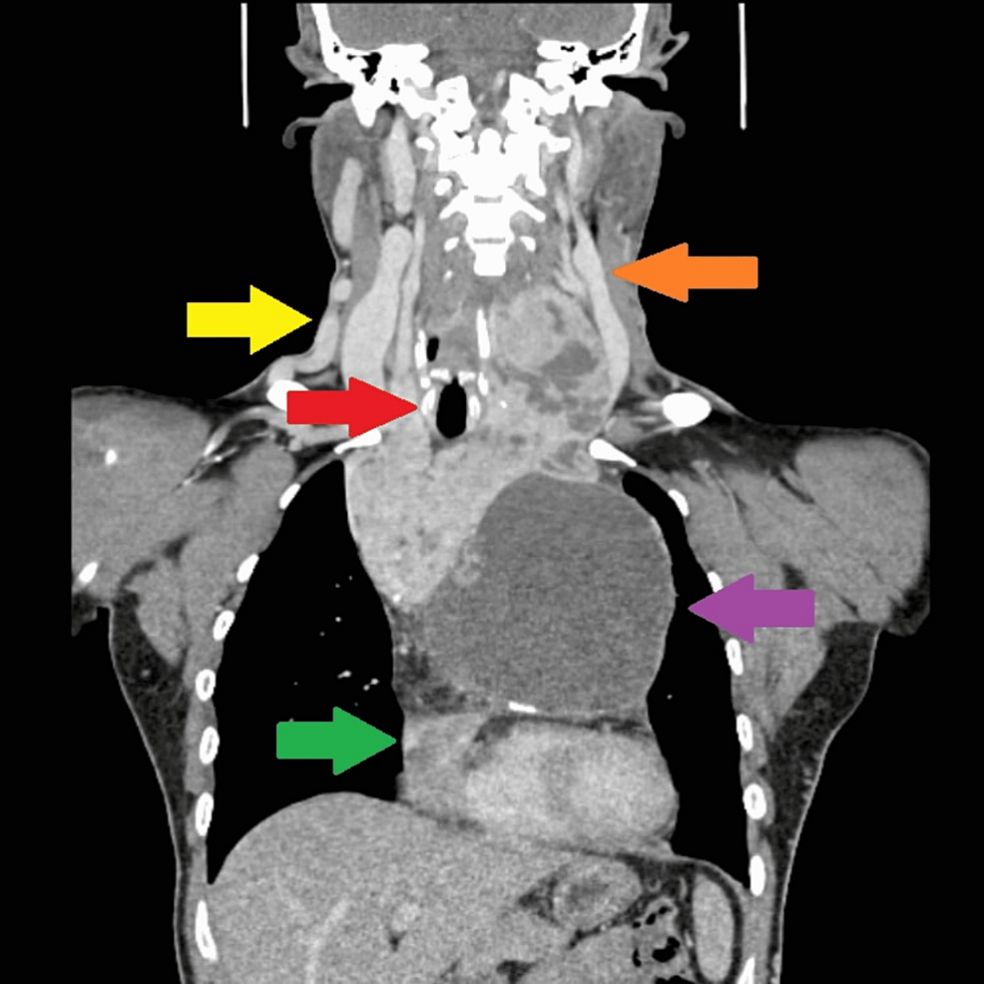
CT (coronal plane) of the thyroid gland with extension into the mediastinum. Yellow arrow indicates dilated right proximal external and internal jugular vein, orange arrow indicates dilated left internal carotid artery, red arrow indicates displaced trachea to right secondary to mass effect of goiter, purple arrow indicates cystic part of the goiter in the mediastinum compressing the heart, and green arrow indicates the compressed heart with clear plane between the pericardium and goiter.
